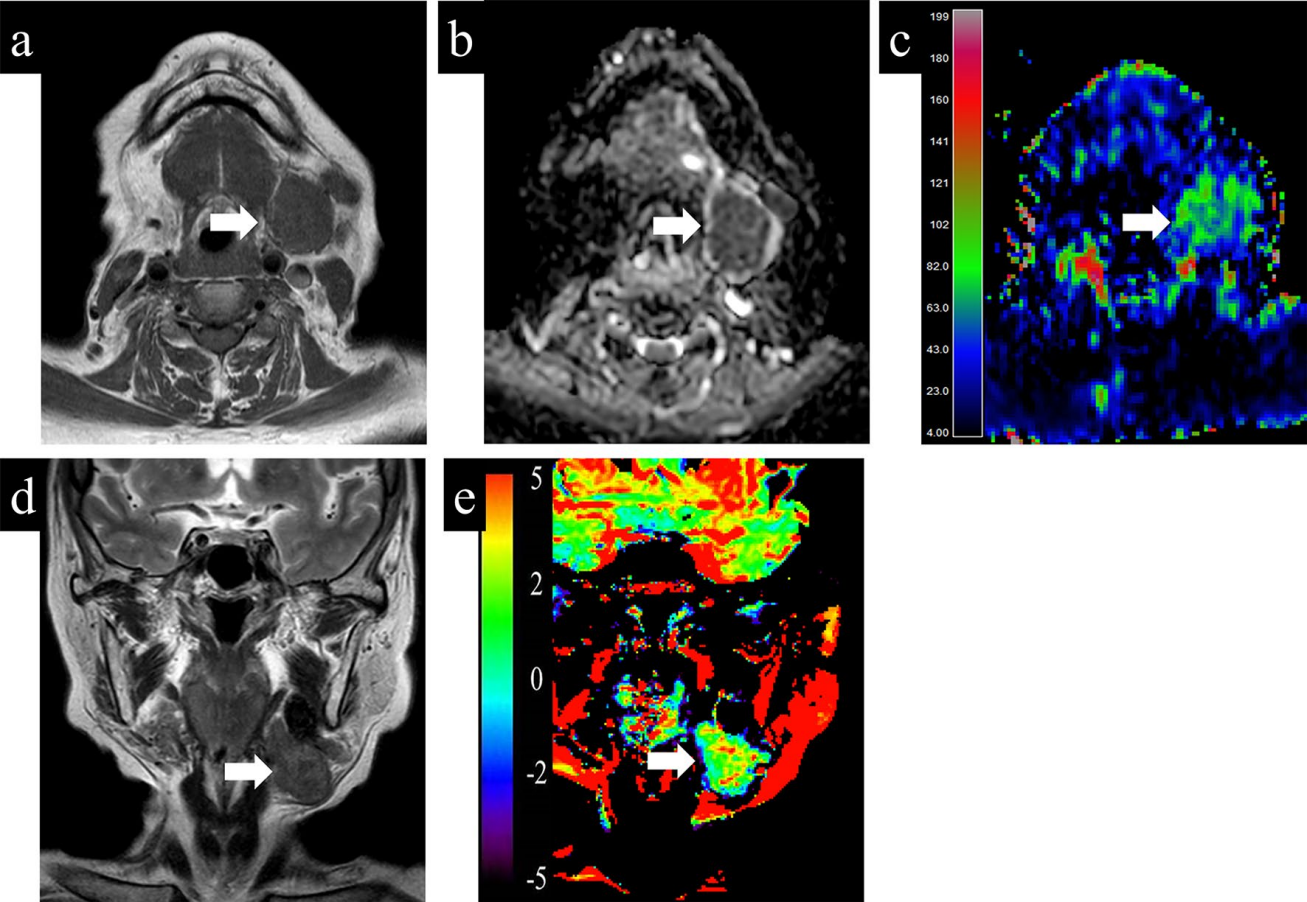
A 75-year-old female with carcinoma ex pleomorphic adenoma. (a) T1-weighted image shows a homogeneous low intensity lesion in the left parotid gland. Apparent diffusion coefficient (ADC) (b) and tumor blood flow (TBF) (c) maps show the lesion with the mean ADC value of 0.91 × 10^−3^ mm^2^/s and the mean TBF value of 67.96 mL/100 g/min, respectively. On T2-weighted coronal image (d), the lesion shows slightly higher signal intensity compared to muscle structure. Amide proton transfer (APT) (e) map shows the lesion with the mean APT signal intensity of 2.65%. An arrow in each image indicates the lesion.
